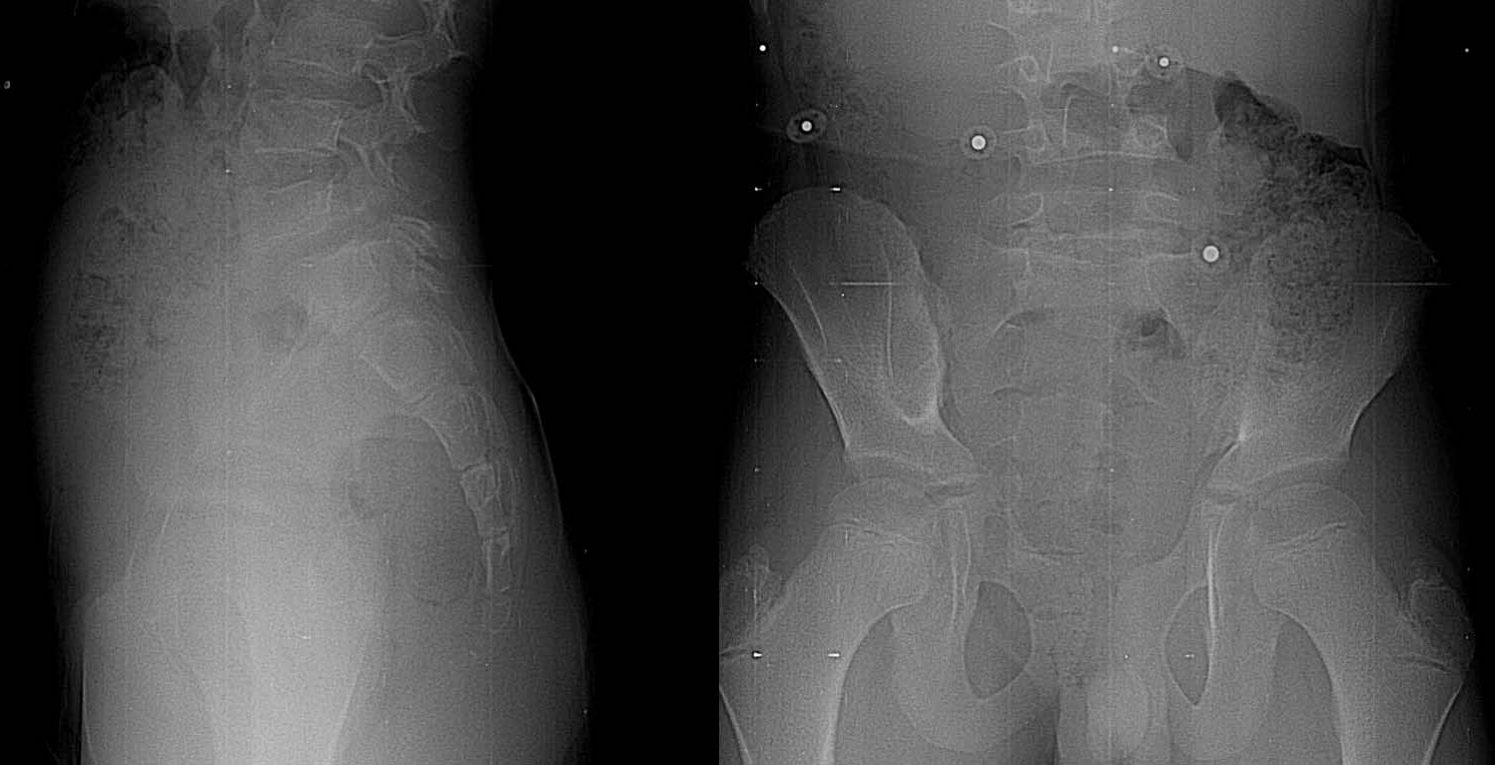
A case of low-grade spondylolisthesis for which there was intra-observer agreement in five of six observers with respect to the degree of dysplasia. However, the inter-observer agreement in the determination of the degree of dysplasia was poor because three observers classified the case as a type I (low-dysplastic), while the three other observers classified it as a type III (high-dysplastic) spondylolisthesis. Only the lumbosacral region from the original radiographs is provided.
